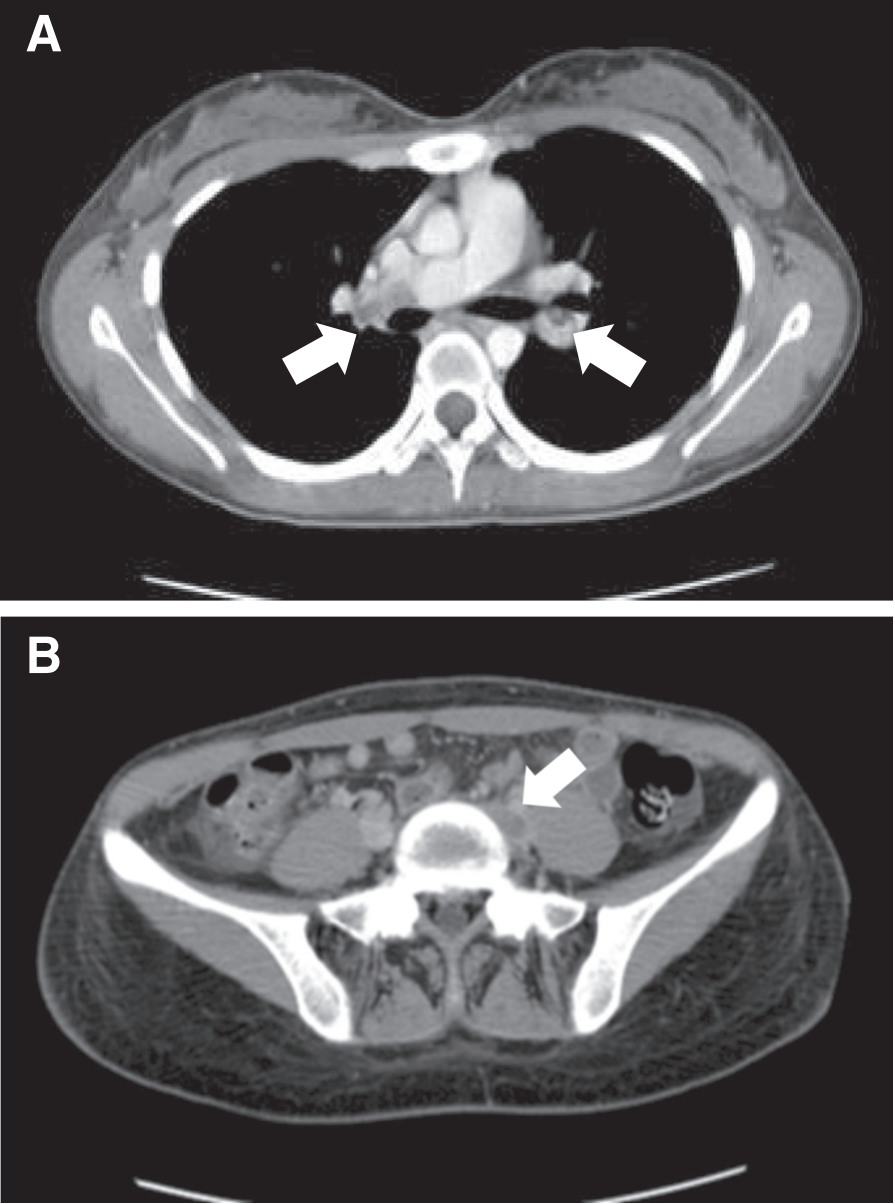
Computed tomography angiogram showing thrombi (arrows) in the pulmonary trunk, bilateral pulmonary artery (A) and left common iliac vein (B).
